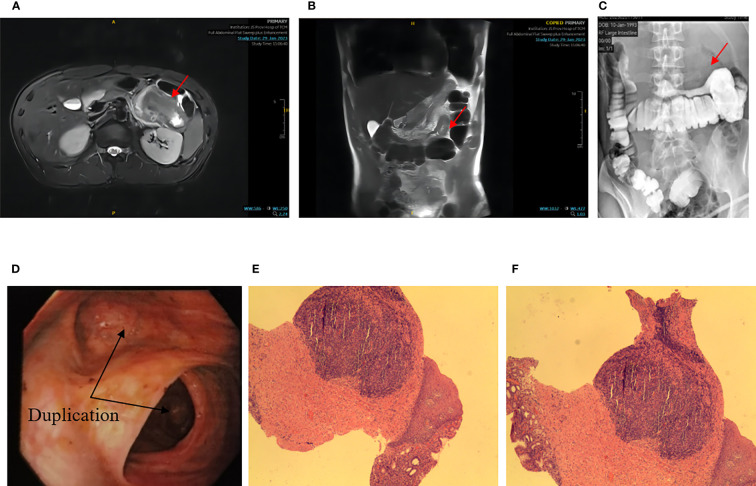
(A) NMR transverse axial view: the red arrow indicates a soft tissue mass located in the left mid-abdomen, closely related to the bowel, approximately 6.4*4.7cm, with multiple internal gas signals; (B) Coronal view of MRI; (C) Barium enema X – ray: the red arrow points to a right-to-left image of a smaller intestinal tube about 190mm long; (D) Endoscopy: the black arrow points to an open-like structure, and the distal segment’s stenosis was obvious; (E, F) Pathology: chronic inflammation of mucosal tissue covered with squamous epithelium and gastric columnar epithelium). Coloration HE, magnification ×100.
